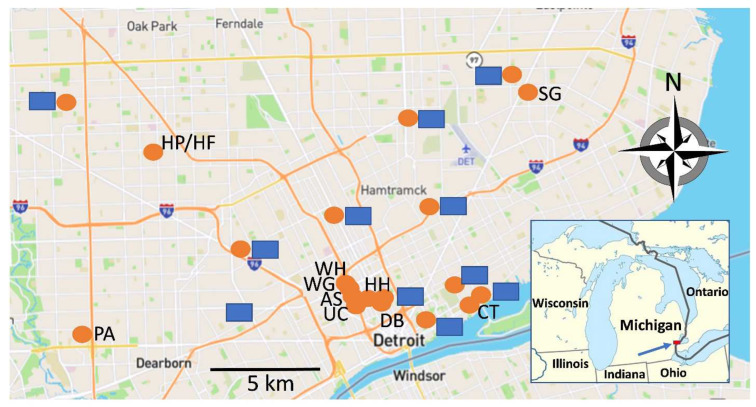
Wastewater sampling sites in Detroit, MI. The blue arrow and red marker in the inset show the location of the site map in Michigan. Collection sites are represented by orange circles, accompanied by either a black label or blue rectangle that obscures the site label for ethical considerations described in the text. Sites sampled for the EGLE-supported pilot project (2020–2021) are Wayne State dormitories, labeled WH, WG, AS, and UC, and adjacent dorm-sampling sites on campus not shown on the map; and hospital sewersheds HH and DB. The subsequent MDHHS-supported project (2021–2023) monitors the four labeled Wayne State sites and two hospital sites; several “ZIP Code” sites labeled PA, HP/HF (see text for explanation of the dual label), CT and SG; and small sewersheds that drain from long-term care facilities and surrounding neighborhoods.
